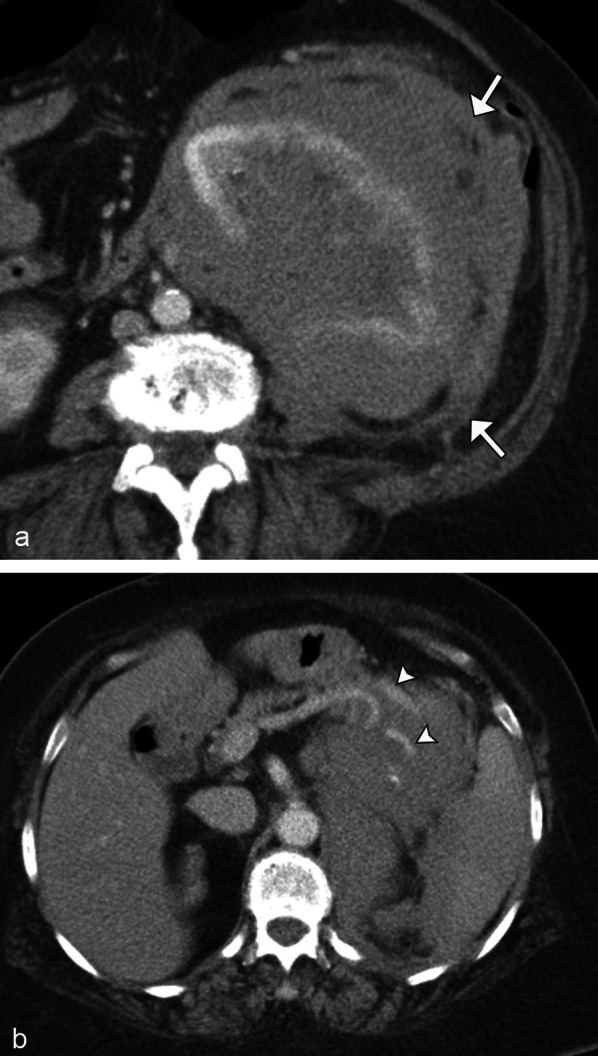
Perinephric space infiltration of renal lymphoma*. Axial CT of the abdomen (a) demonstrates an infiltrative homogenous mass (arrows) in the left retroperitoneum enveloping the left kidney with preservation of the left renal parenchymal anatomy. Additional thickening of Gerota’s fascia and Zuckerkandl fascia as well as retroperitoneal tumor infiltration along the pancreatic tail and splenic hilum are present (b). Note the preservation of normal caliber splenic vein and artery (arrowheads). Due to the unusual tumor morphology, the left renal mass was biopsied and consistent with diffuse large B-cell lymphoma
